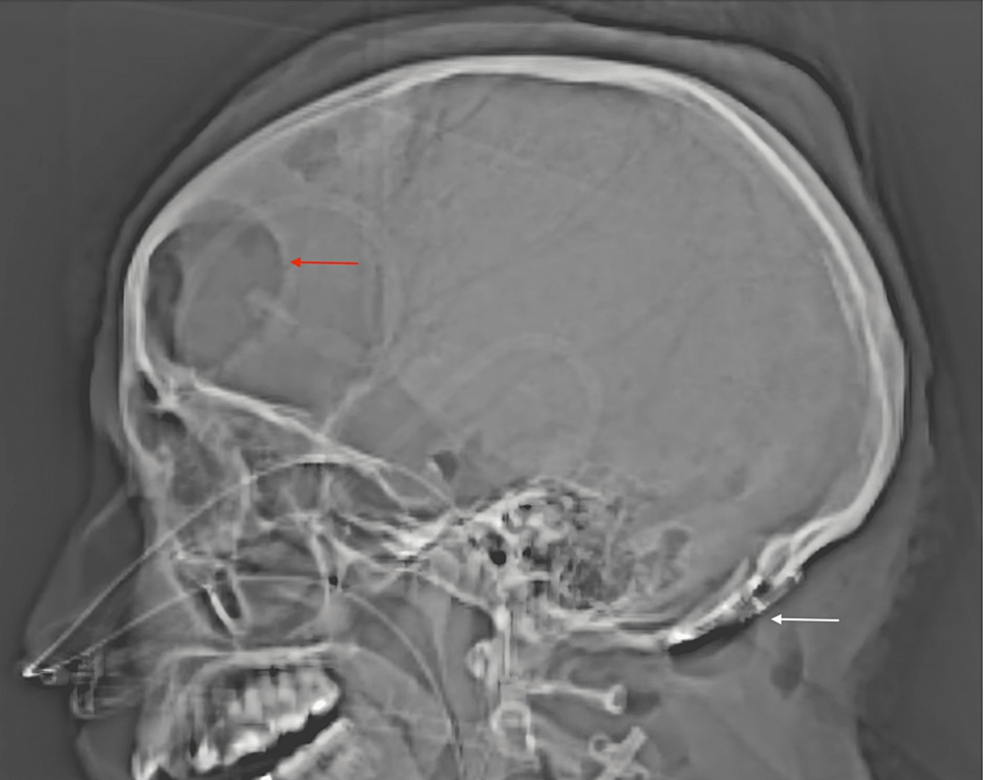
CT head scout image demonstrates the removal of the bullet from the fourth ventricle through a suboccipital craniotomy (white arrow). The external ventricular drain has been removed. Red arrow = frontal craniotomy.
